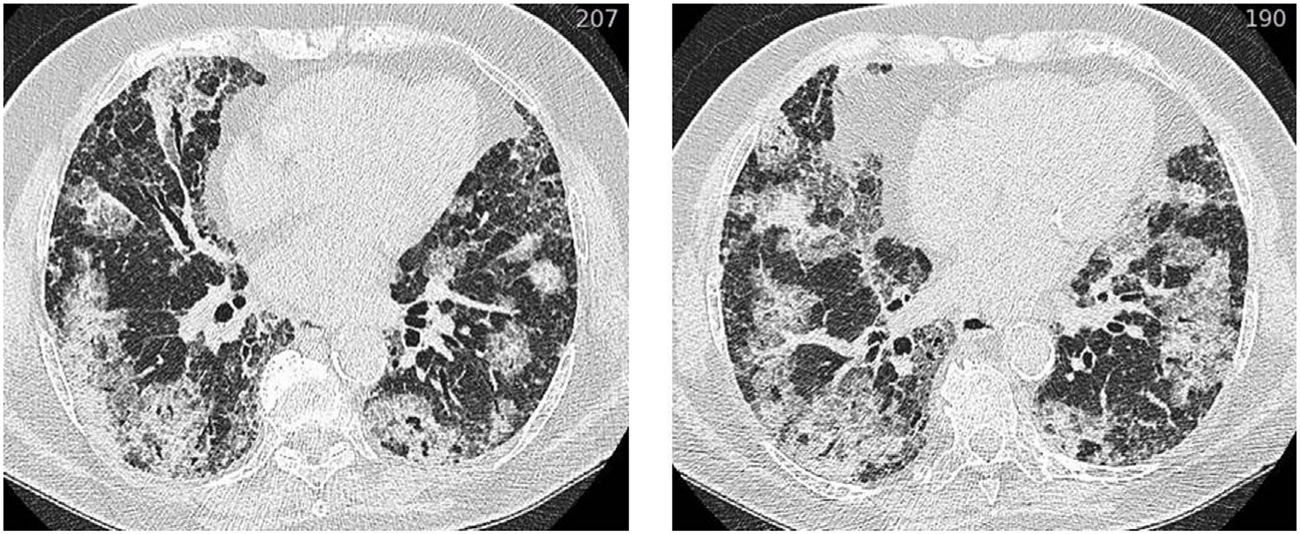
HRCT of an acute exacerbation in IPF. Axial HRCT of a patient with IPF at the time of an acute exacerbation shows extensive bilateral ground-glass opacification. Abbreviation: HRCT, high resolution computed tomography; IPF, idiopathic pulmonary fibrosis.
